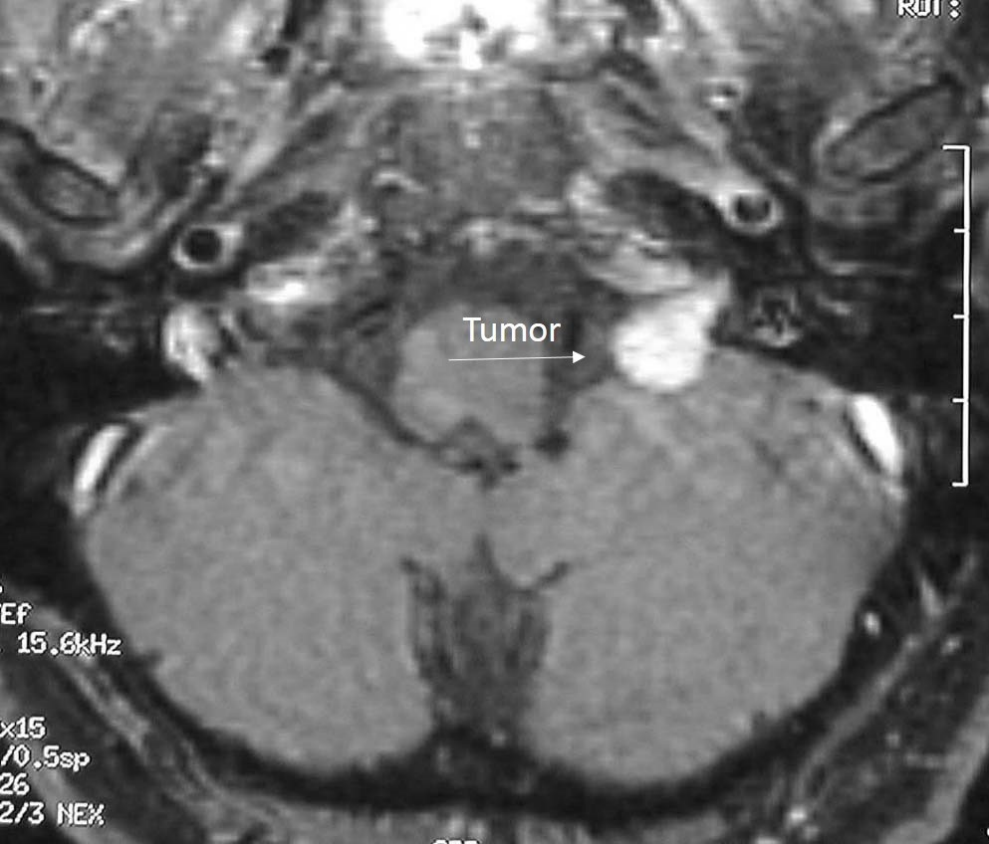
Preoperative MRI of Case 1 Preoperative MRI depicting a homogenously enhancing lesion originating from the jugular foramen.
